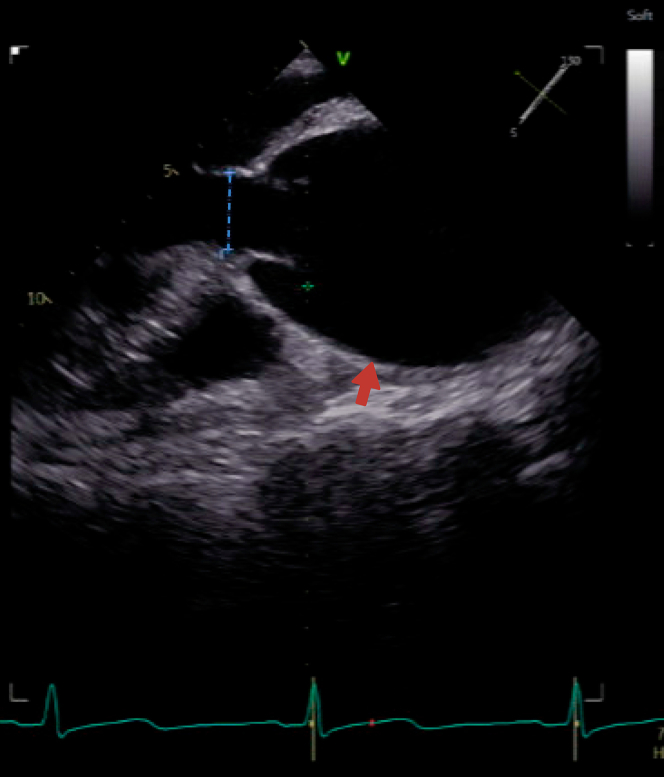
Preoperative Echocardiogram Parasternal long-axis view demonstrating a dilated aortic root and ascending aortic aneurysm (red arrow). The aortic annulus is indicated (blue dotted line).
